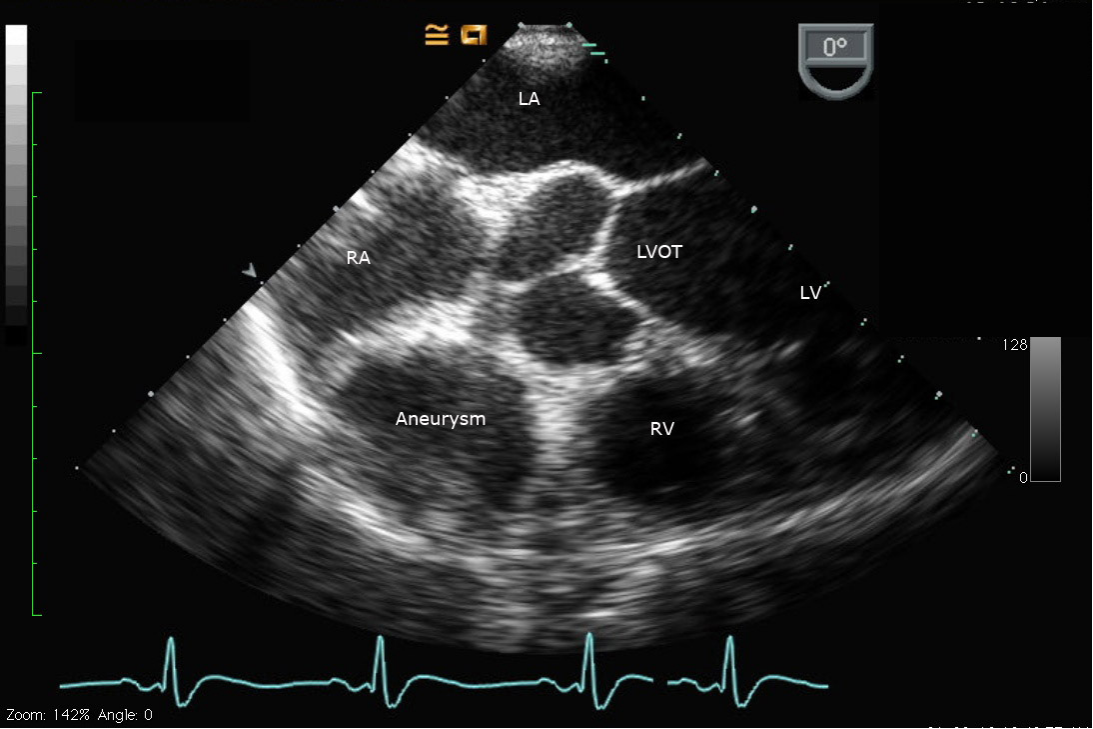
Midesophageal transesophageal modified short axis view of the right ventricular outflow tract. RA - right atrium; RV - right ventricle; LV - left ventricle; LVOT - left ventricular outflow tract.
